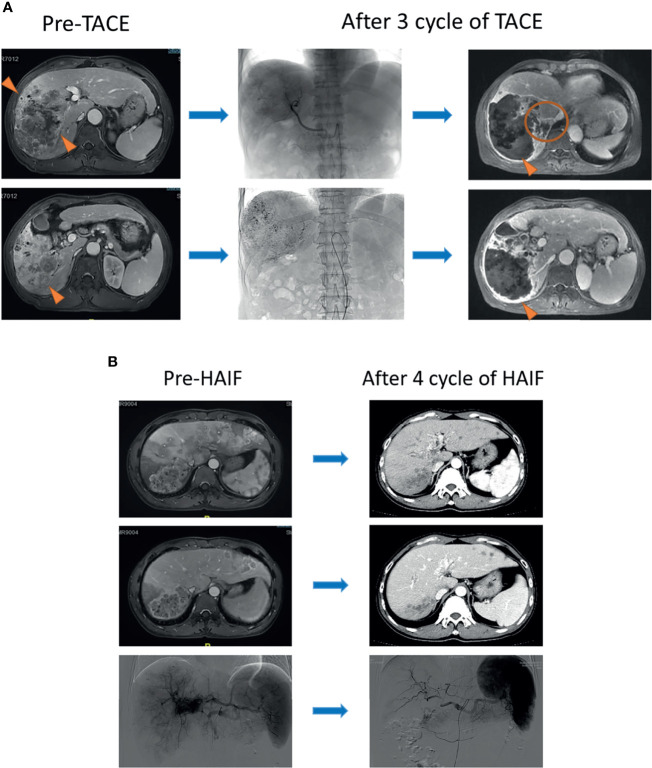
(A) A 57-year-old male patient was diagnosed with infiltrative HCC by contrast MRI scanning and received three-cycle TACE. Obvious necrosis appeared in the center of the tumor after therapy (triangle), but the marginal lesions became enlarged homogeneously (circle). (B) A 46-year-old male patient with multiple lesions was diagnosed with infiltrative HCC. Portal vein fistula was revealed at the arterial phase of the MRI scanning image. After a four-cycle HAIC, all of the lesions in the liver apparently shrank (triangle).
